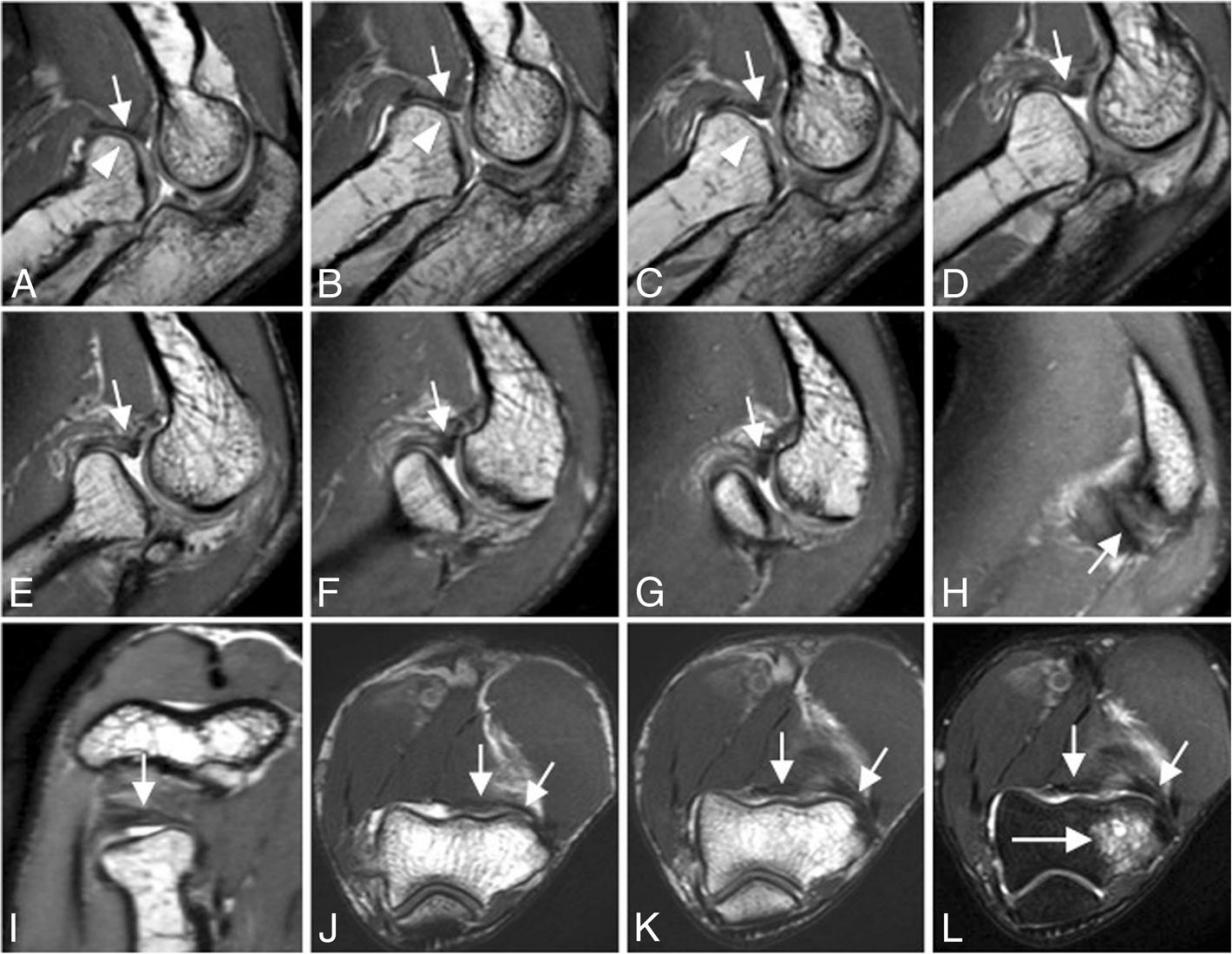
A 46-year-old man with recurrent painful click in the elbow. Consecutive sagittal 3D-FSE PD-weighted MRI (a to h), consecutive axial 3D-FSE PD-weighted (i to k), and axial FS PD-weighted MRI (l) show superior displacement of annular ligament interposing between the radial head and the capitellum (white short arrows). Radial head deformity (white arrowheads). Capitellar osteochondral lesion (long white arrow)
